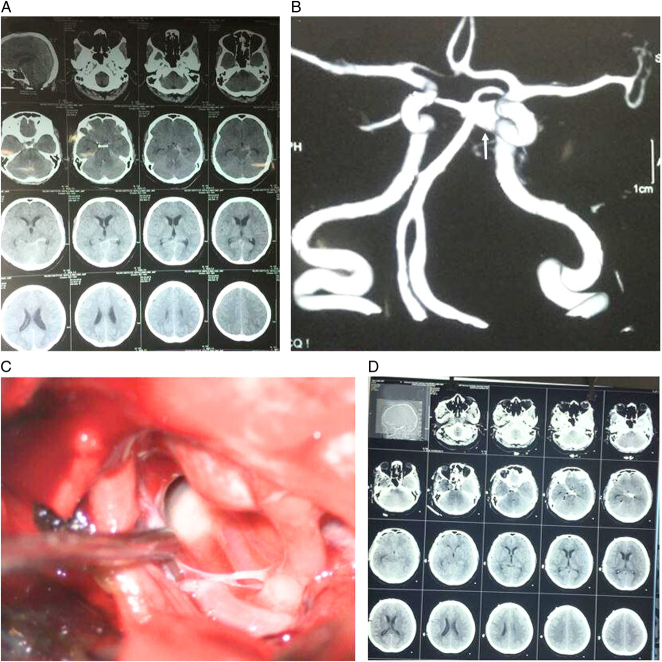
Imaging studies of a 55-year-old woman presenting in the Hunt and Hess grade IV. (A) Plain CT scan shows diffuse subarachnoid haemorrhage. (B) CT angiography reformatted view showing a basilar tip aneurysm with the dome projecting toward the left (arrow). (C) Intraoperative picture showing atherosclerosis of the entire aneurysm, and (D) postoperative CT showing diffuse low density in the brain stem. The patient died 6 days after surgery. CT, computed tomography.
